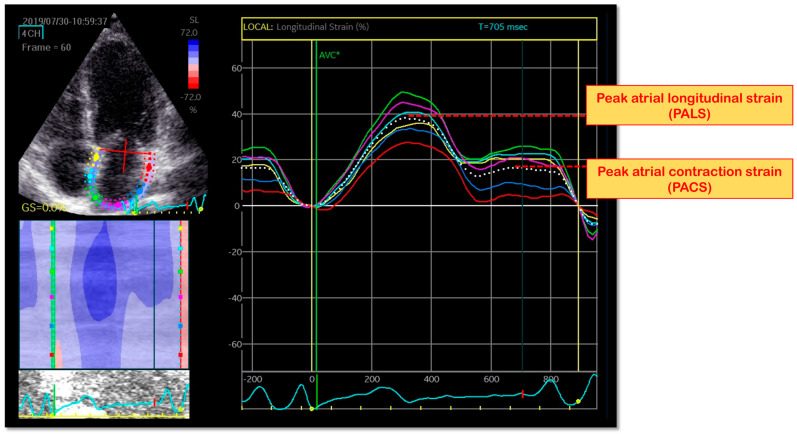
Left atrial strain parameters by speckle-tracking echocardiography.
